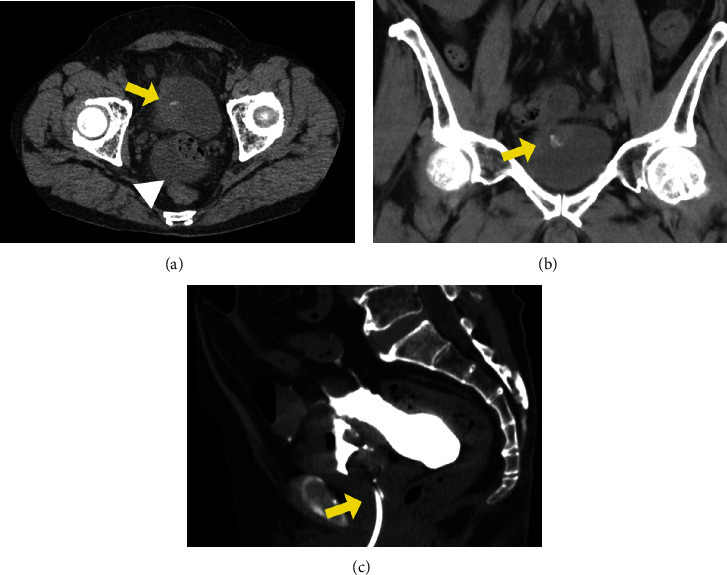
(a) An axial plain abdominal computed tomography (CT) scan showed bladder wall thickening with calcification (arrow) and ascites in the rectovesical pouch (white arrowhead). (b) A coronal plain abdominal CT scan showed bladder wall thickening adjacent to the ileum (arrow). (c) A sagittal CT scan after intravesical injection of contrast medium via a urethral catheter (arrow) showed leakage of contrast medium into the abdominal cavity.
